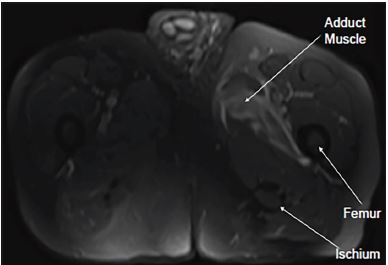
Magnetic resonance imaging demonstrating acute myositis of the left proximal adductor muscles.
